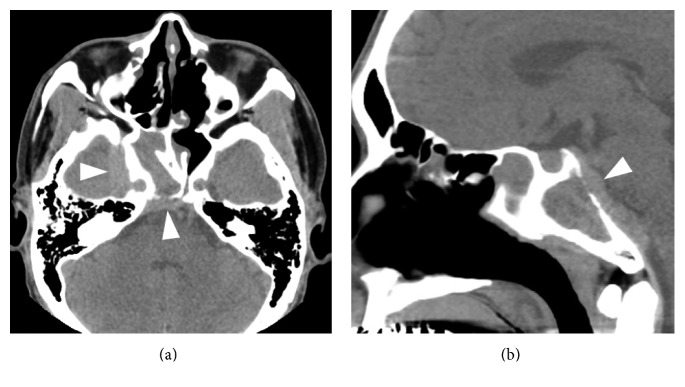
CT scan 18 months after being diagnosed with GPA shows a lesion of the right sphenoid sinus eroding the bone of the clivus and thickening of the dura mater on axial (a) and sagittal (b) images (triangle).
